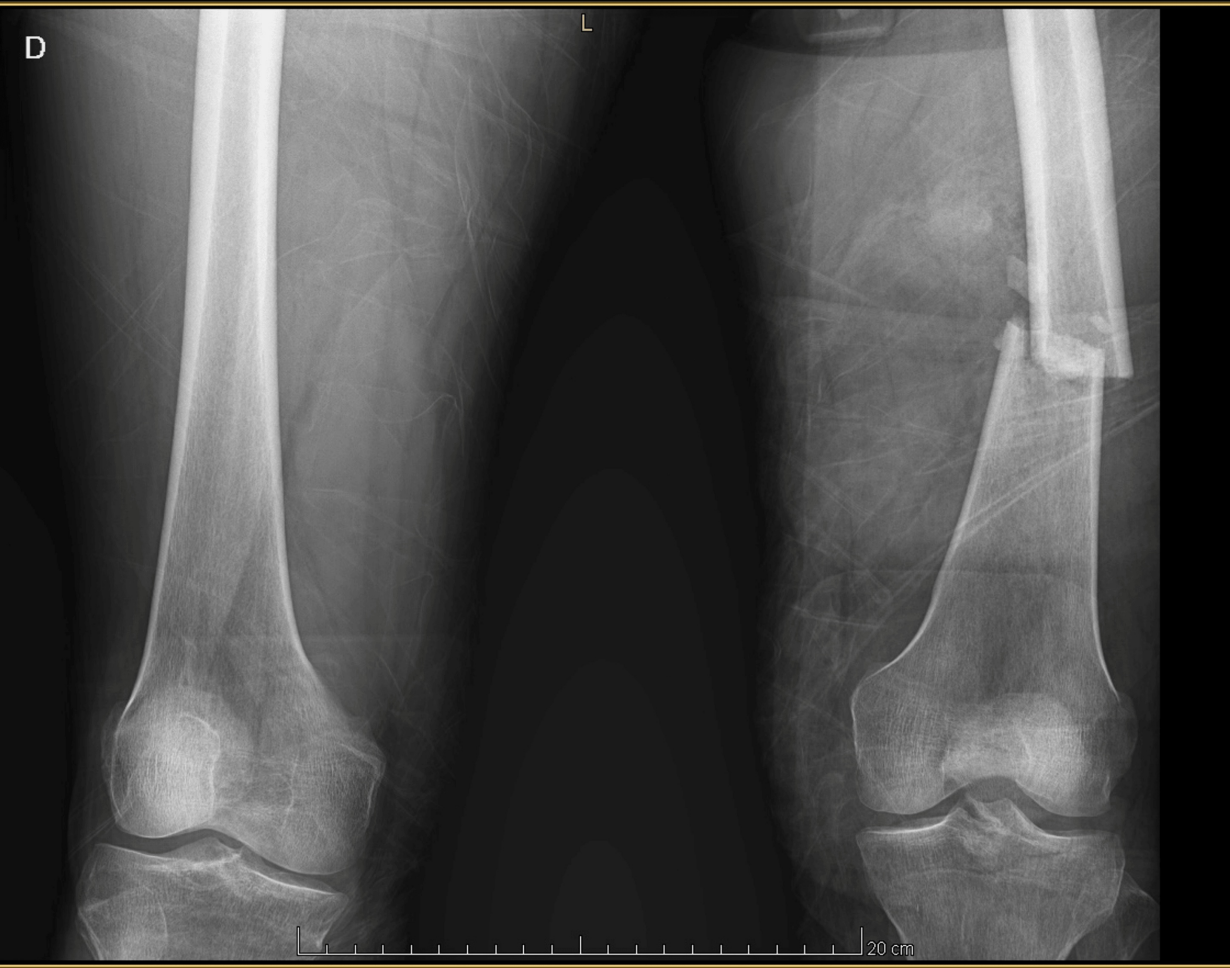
Bilateral femur X-ray showing a left femoral fracture. Anteroposterior radiograph of both femurs showing a transverse fracture of the midshaft of the left femur, without associated displacement. This injury was part of a multiple trauma scenario and contributed to the development of acute compartment syndrome.
